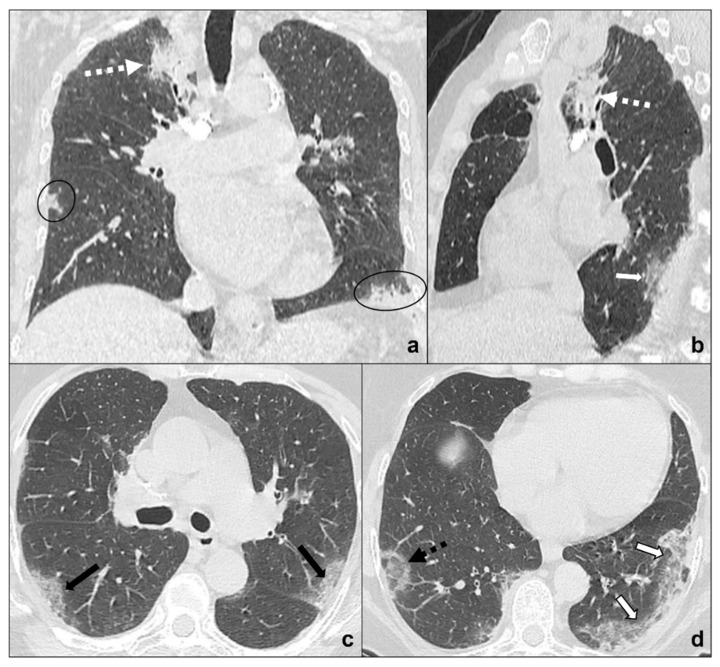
(a–d) Chest CT of an oncologic patient affected by a paramediastinal lung cancer located in the apical segment of the right upper lobe (dotted white arrow) and undergoing chemotherapy and radiotherapy. After admission to the first aid with ageusia, anosmia, and fever, a chest CT scan was performed showing subpleural areas of ground-glass (white arrow in (b)) and crazy paving (black arrow in (c)) with the typical reversed halo sign or atoll sign (dotted black arrow in (d)), parenchymal consolidations (black circle in (a)) and subpleural parenchymal bands together with crazy paving opacities (black-bordered white arrow in (d)). Lungs: mean window with 1500 HU; mean window level −600. Mediastinum: mean window with 350 HU; mean window level 50 HU.
